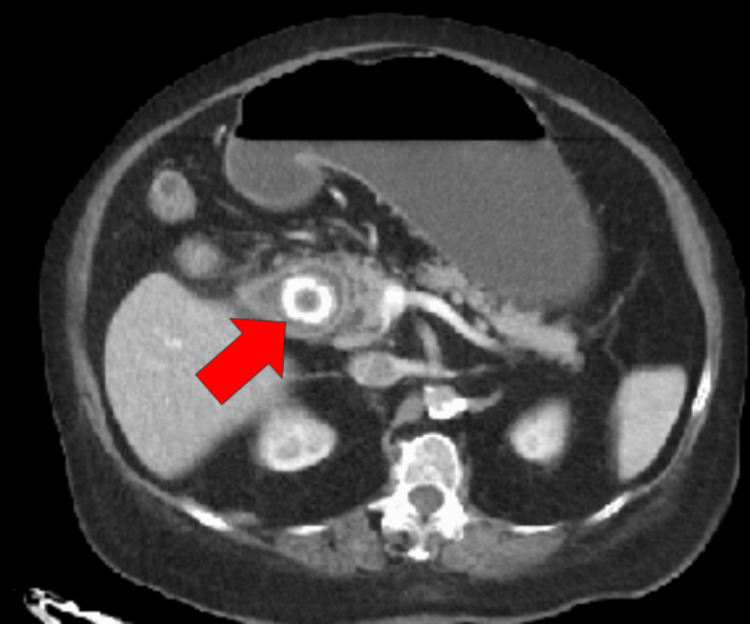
Contrast-enhanced abdominal-pelvic computed tomography (CT) image (axial view). This image demonstrates a large gallstone obstructing the proximal duodenum via a cholecystoduodenal fistula.
